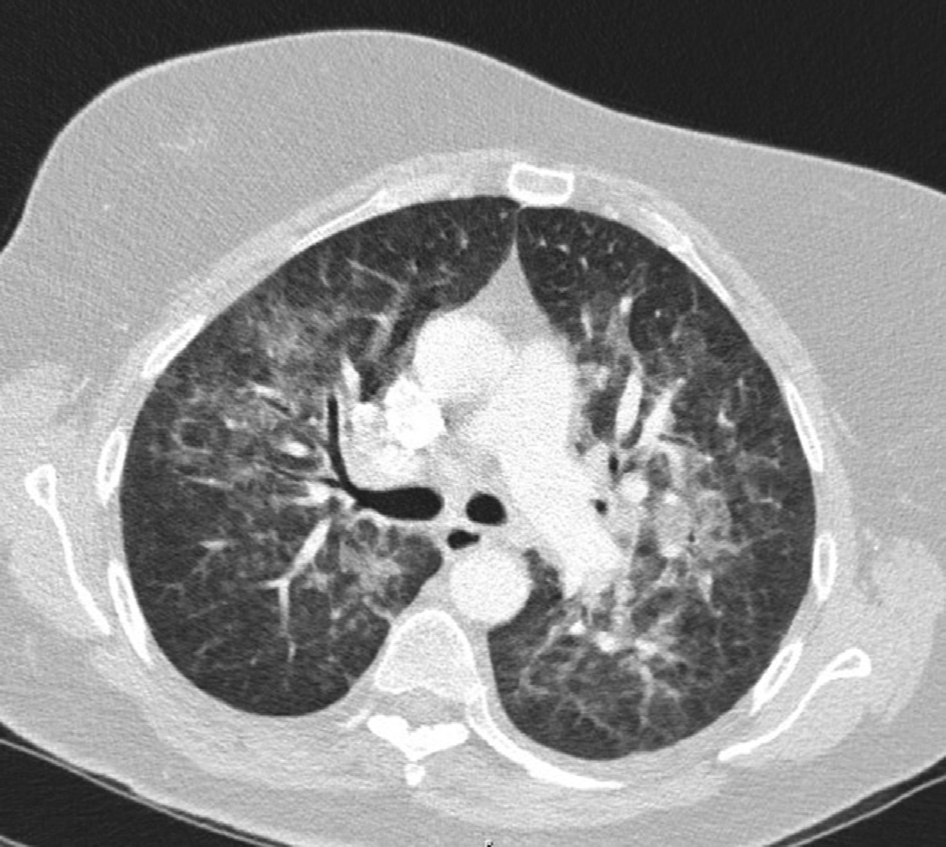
CT imaging of the thorax of patient 1 showing interstitial pulmonary infiltrates.
